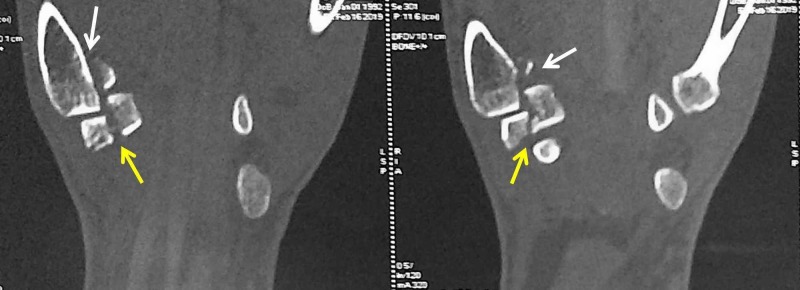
Computed tomography (coronal sections) showing Bennett fracture and trapezium fracture White arrows - Bennett fracture; yellow arrows - trapezium fracture
